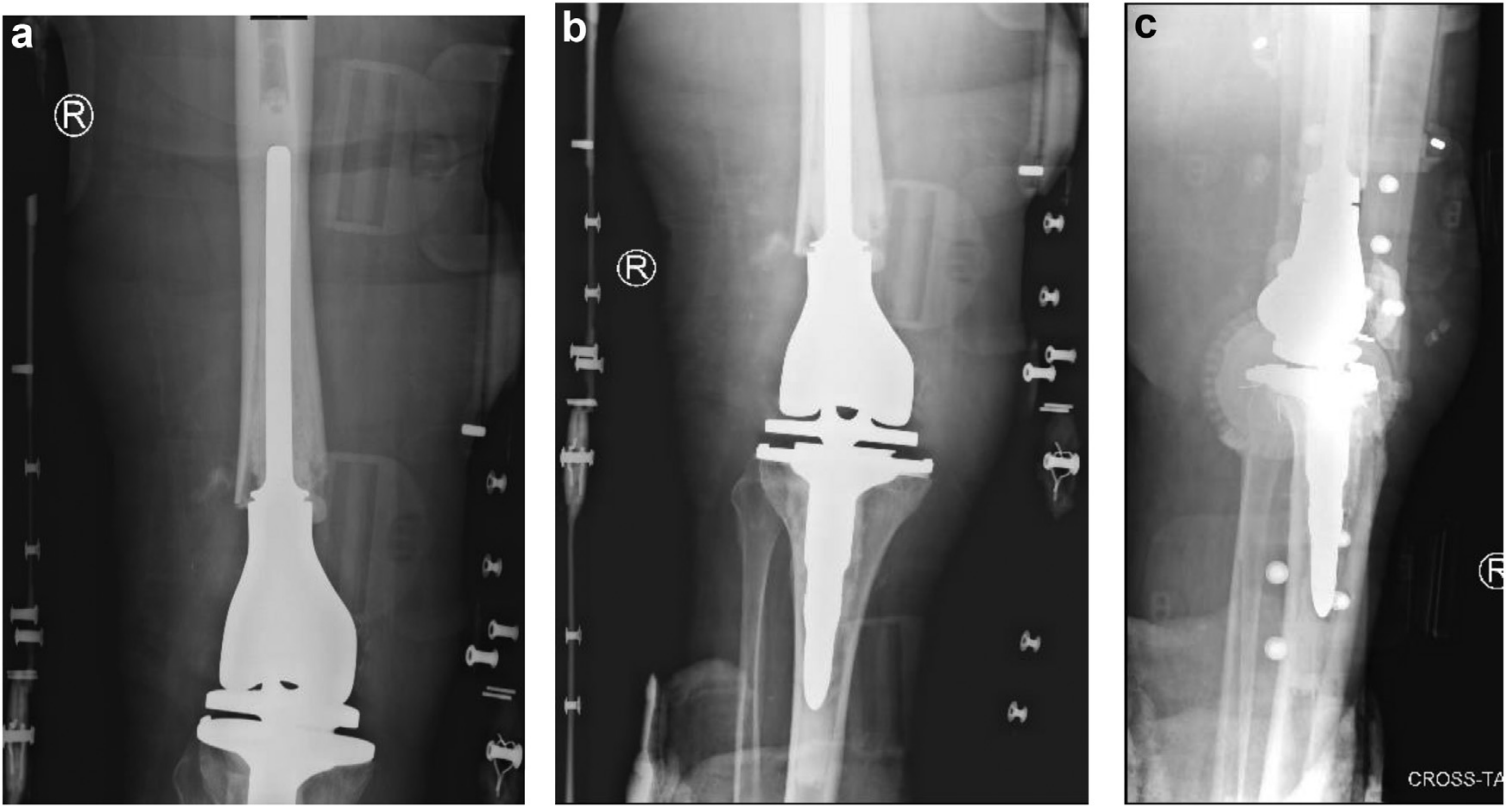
Anteroposterior (a and b) and lateral (c) 2-week postoperative radiographs demonstrating cemented distal femoral replacement with maintenance of alignment, rotation, and fixation. Insall-Salvati ratio of 1.18.
